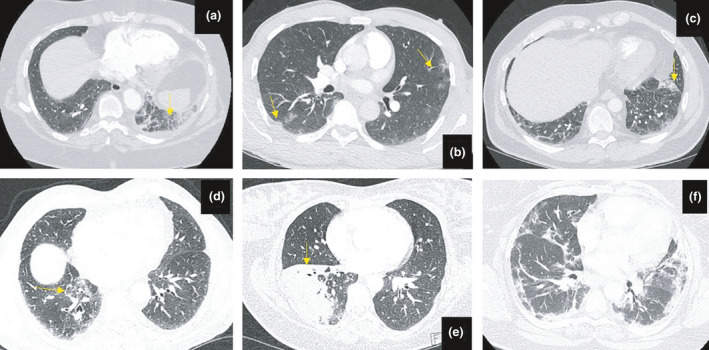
Examples of lung parenchymal changes on CT pulmonary angiogram (CTPA). Parenchymal changes are indicated by the yellow arrows. a = 2020 CTPA positive for PE with associated parenchymal changes [ground glass opacities (GGOs)]; b = 2020 CTPA negative for PE with multiple peripherally distributed GGOs; c = 2020 CTPA negative for PE with a single peripheral GGO; d = 2019 CTPA negative for PE with peri‐bronchial GGOs; e = 2020 CTPA negative for PE with right lower lobe consolidation; f = an example of more extensive parenchymal changes in a patient who had a positive PCR test for COVID‐19.
